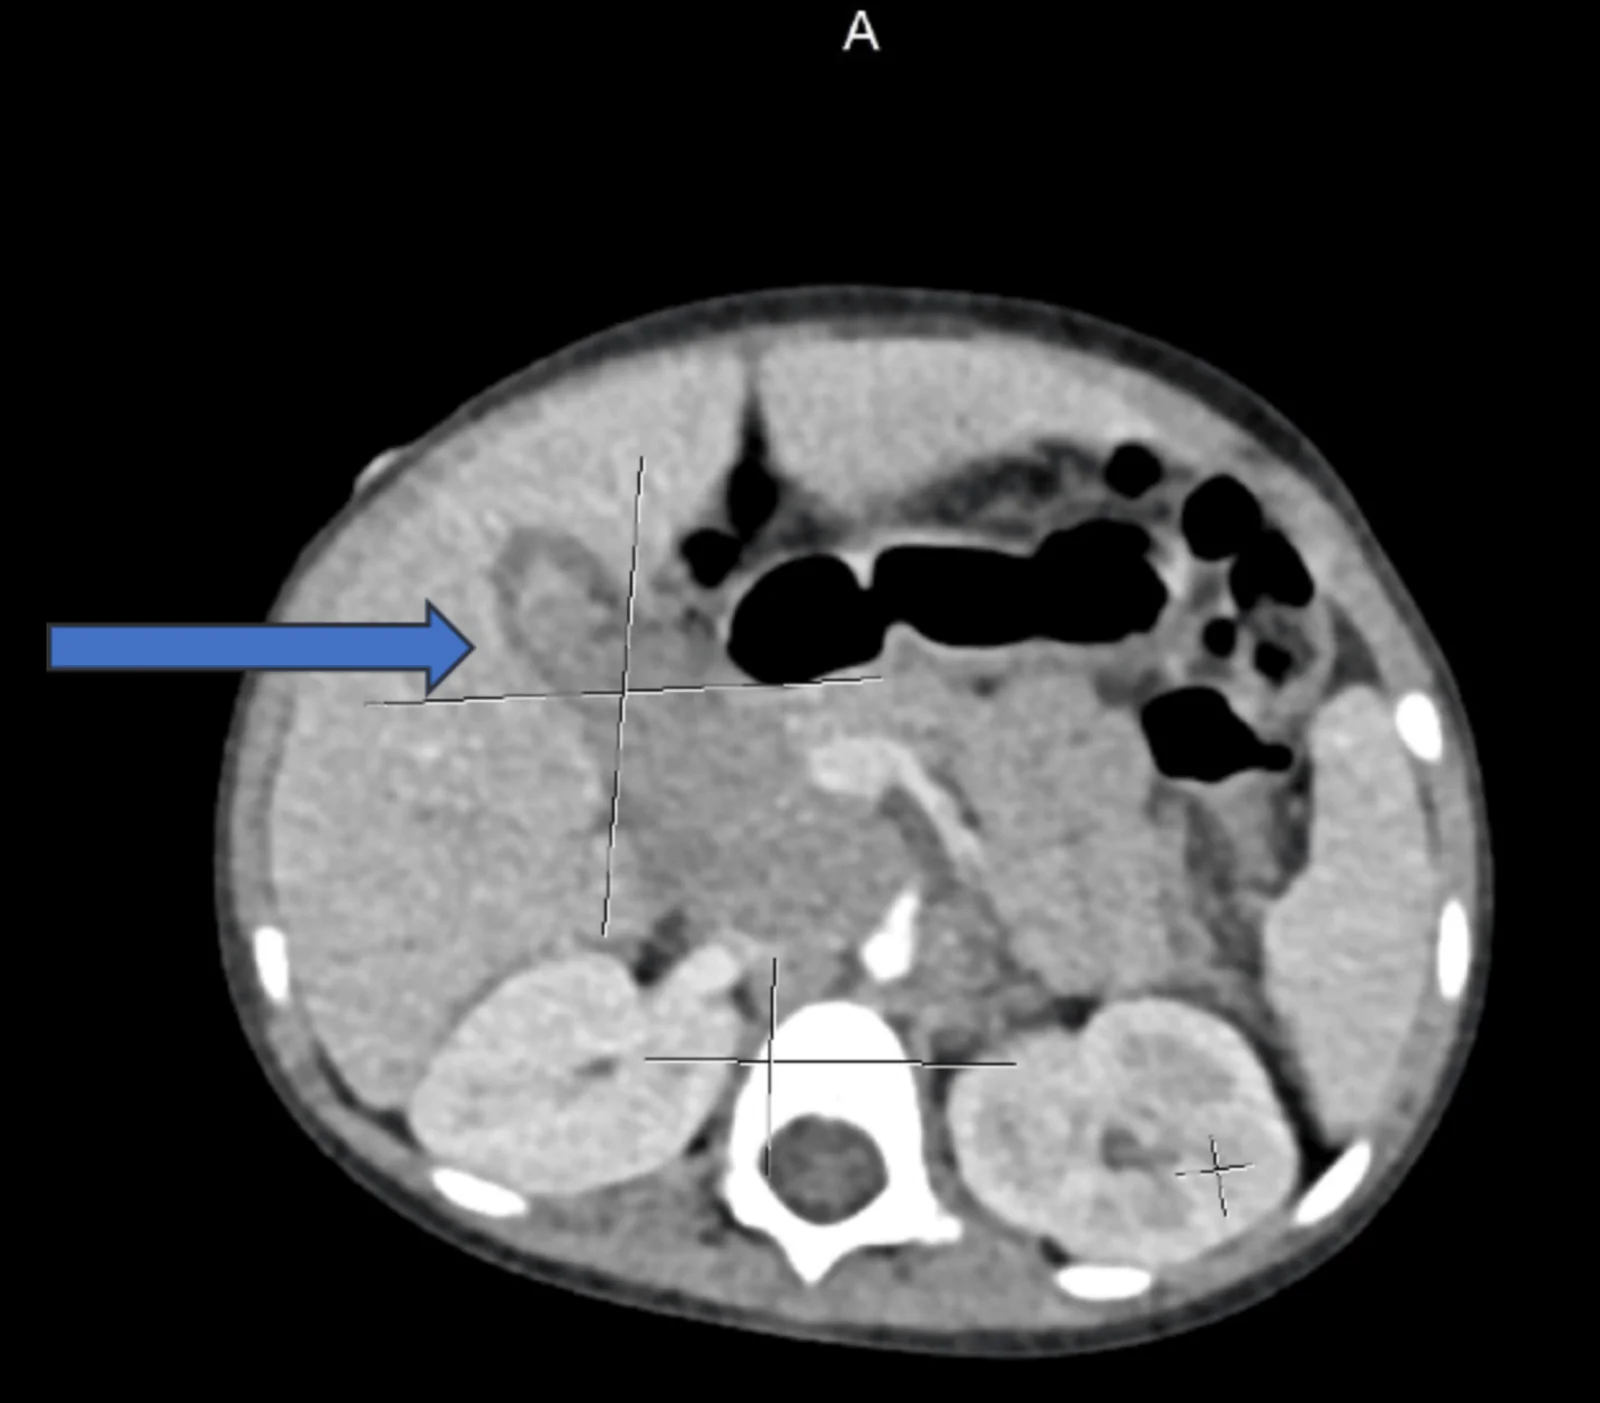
Contrast-enhanced CT scan demonstrating the porta hepatis mass during inpatient hospitalization CT: computed tomography
